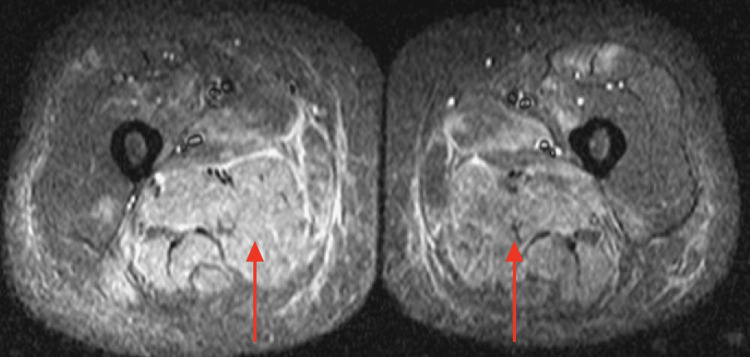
MRI of Bilateral Legs Revealing Edema Within the Muscles Increased signaling in the bilateral adductors, indicated with red arrows, as well as the right semimembranosus and semitendinosus, indicating edema within the muscles.
